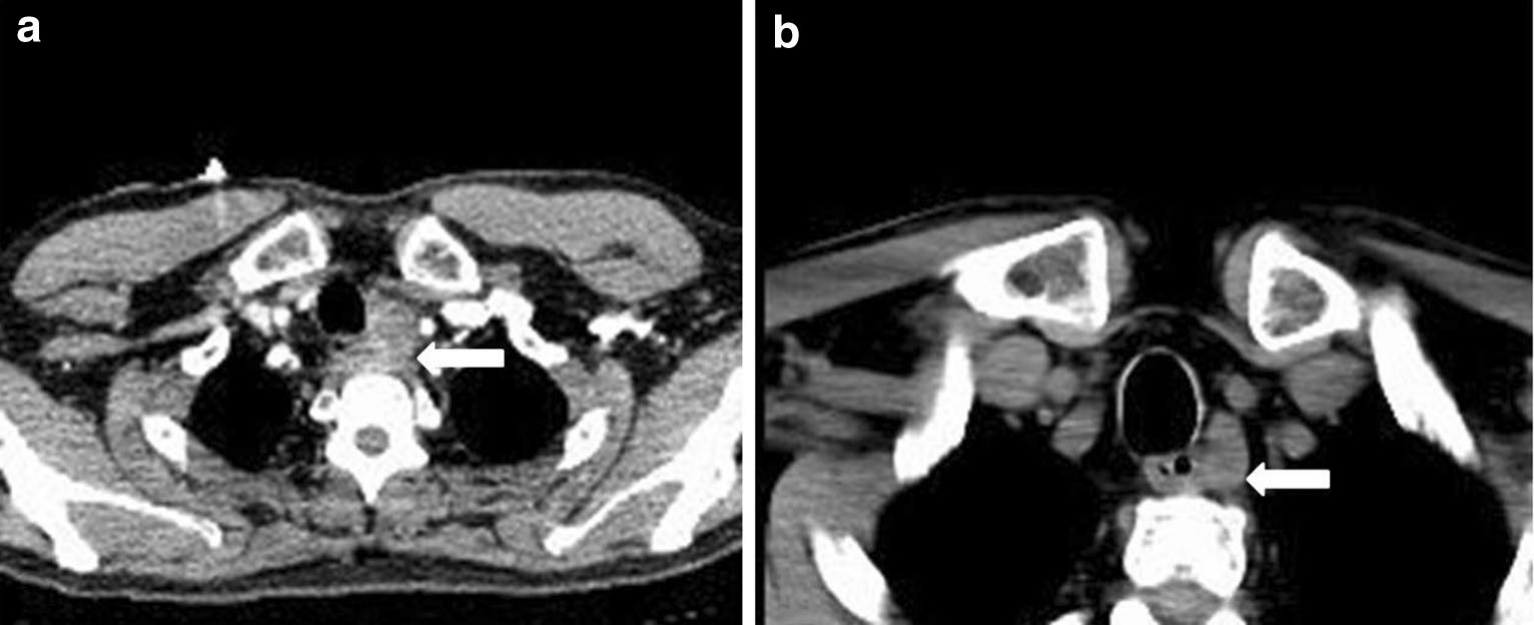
Case 2. a Axial image of a CT of the neck and chest with contrast demonstrate diffuse soft tissue density (*arrow*) in the posterior mediastinal space. b Axial image of a CT of the neck and chest without contrast examined 2 weeks after admission. The diffuse lesion has disappeared and a mass (*arrow*) posterior to the thyroid can be clearly seen
